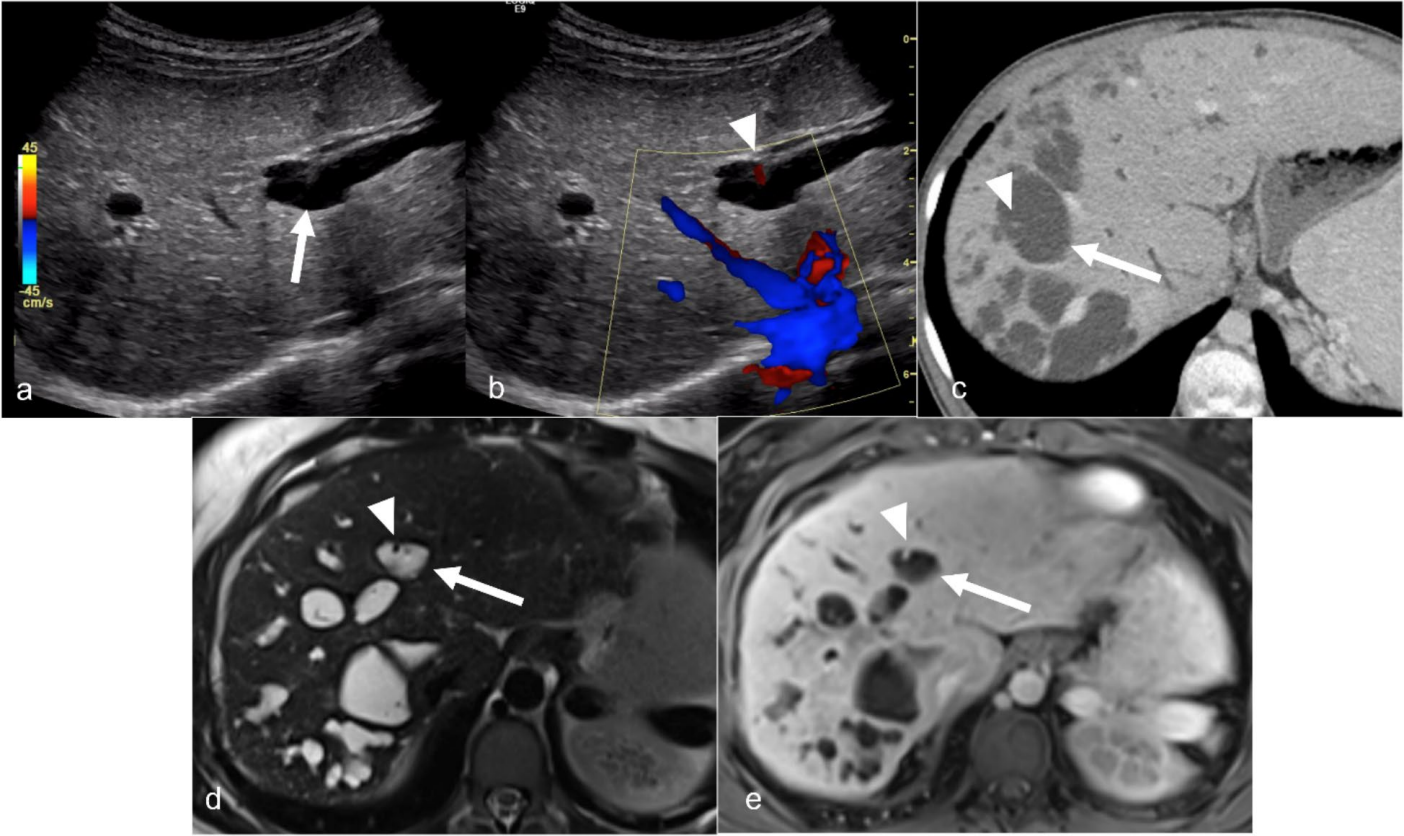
Caroli disease in a 39-year-old patient with abdominal pain. Grayscale (a) and color Doppler (b) ultrasound images show a dilated bile duct (arrow) with a dot of blood flow (arrowhead). Axial contrast-enhanced CT (c) shows multiple cysts (arrow) with a central dot sign of enhancing portal vein branch (arrowhead). Axial MRI T2-weighted fat-saturated (d) and postcontrast T1 (e) images show multiple T2-weighted hyperintense cysts (arrow) with a small focal area of hypointensity (arrowhead), which shows enhancement, corresponding to a portal vein branch on postcontrast image (arrowhead, e)
